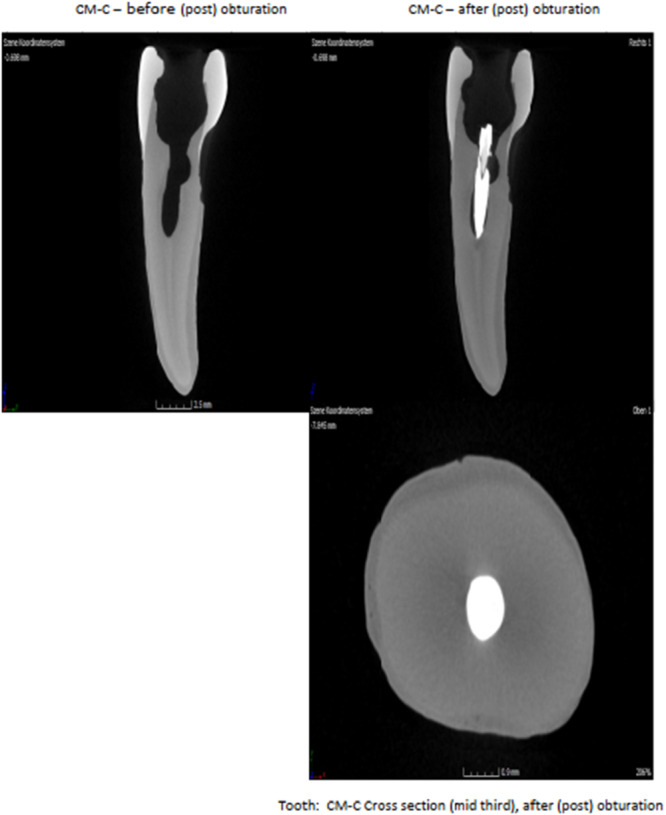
CT Images of teeth obturated.
